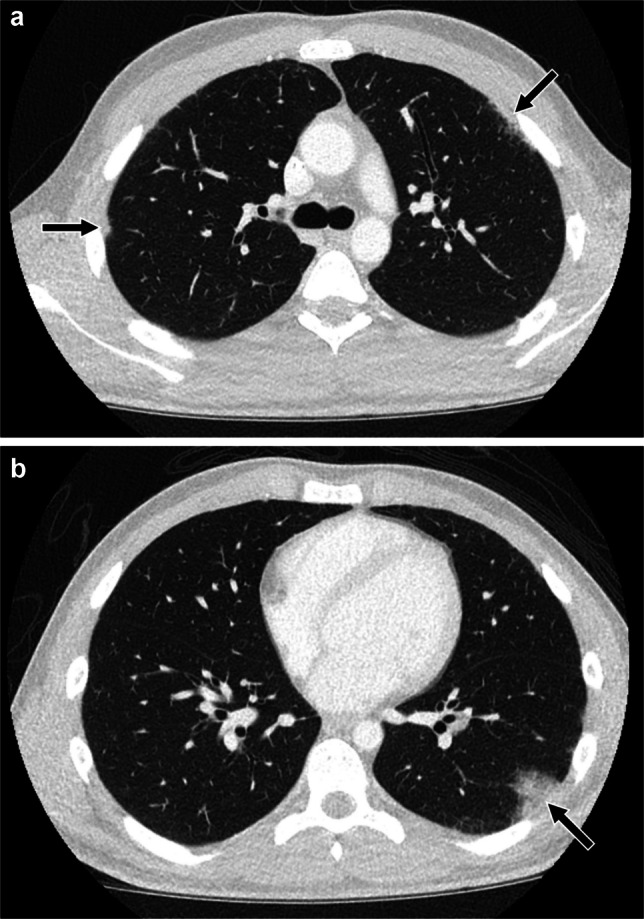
A 17-year-old male who developed exercise intolerance and progressive decline in diffusion capacity on pulmonary function testing during the course of several cycles of chemotherapy that included bleomycin for testicular mixed germ cell tumor. He smoked marijuana, but this was not a new or changed habit, and his family members smoked cigarettes outside the home. There were no clinical signs or symptoms of infection, and his metastatic disease had been responding to treatment. a-b Axial CT images with contrast showing multifocal bilateral peripheral consolidation (*black arrows*) that could be seen with organizing pneumonia or chronic eosinophilic pneumonia, with lack of peripheral eosinophilia at the time favoring the former. No pulmonary embolus was seen. Clinical and imaging findings were thought to likely relate to bleomycin-induced lung disease, but bleomycin was continued given that the patient only had one more cycle left and his diffusion capacity had not reached the threshold for bleomycin dose adjustment according to protocol. His symptoms and lung imaging findings had resolved 6 months later, after chemotherapy had been completed, and his pulmonary function testing had substantially improved supporting a diagnosis of bleomycin-induced lung disease
